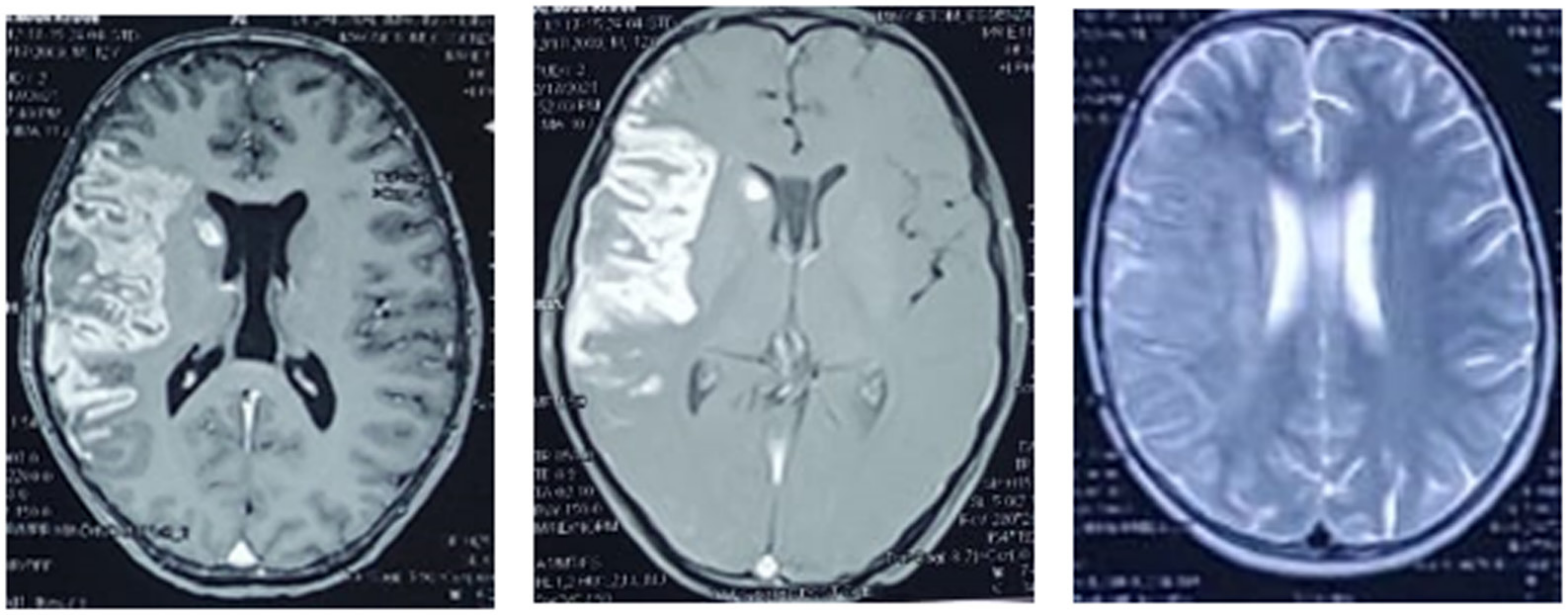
(A–C). MRI Brain showing restriction diffusion at right lentiform nucleus, caudate nucleus, centrum semiovale and corona radiata which appeared hyper‐intense on T2/FLAIR and hypo‐intense on T1 with patchy contrast enhancement in centrum semiovale suggestive of vasculitic infarct. Few T2 hypo‐intense homogeneous enhancing lesions seen in region of caudate nucleus suggestive of granulomatous lesion. Large area of T2/FLAIR hyperintensity in cortical, subcortical and deep white matter of right fronto‐parietal region with heterogeneous contrast enhancement involving the meninges, cortical and subcortical regions with area of FLAIR sulcal hyperintensity suggestive of meningoencephalitis.
